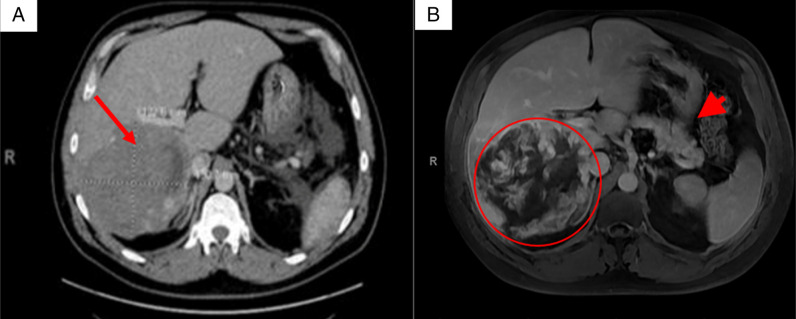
(A) Lobulated, heterogeneous density lesion seen in the right lobe liver, most compatible with a hemangioma. (B) Magnetic resonance imaging axial section T1 fat saturated postcontrast during the venous phase: An 18 cm right hepatic lobe mass with incomplete peripheral nodular enhancement characteristic for hemangioma (circle). A normal pancreas is partially seen (short arrow).
